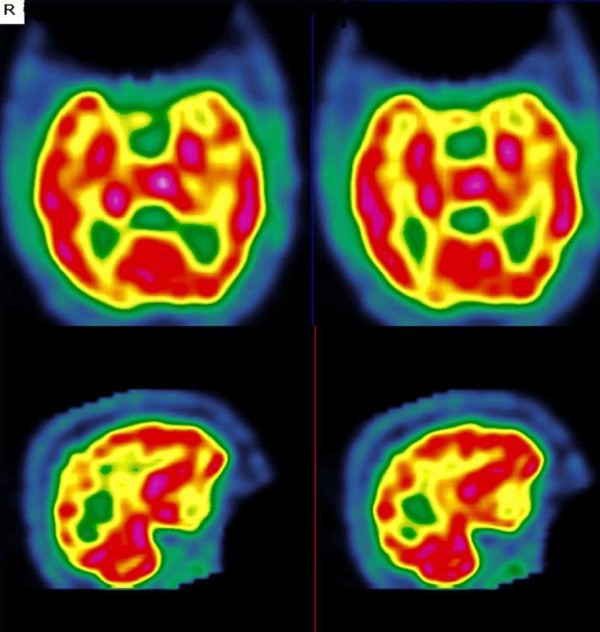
Results from the first SPECT scan (during the course of lenalidomide treatment). Note the left temporal hypoperfusion in the transverse (top) and sagittal (bottom) slices.
